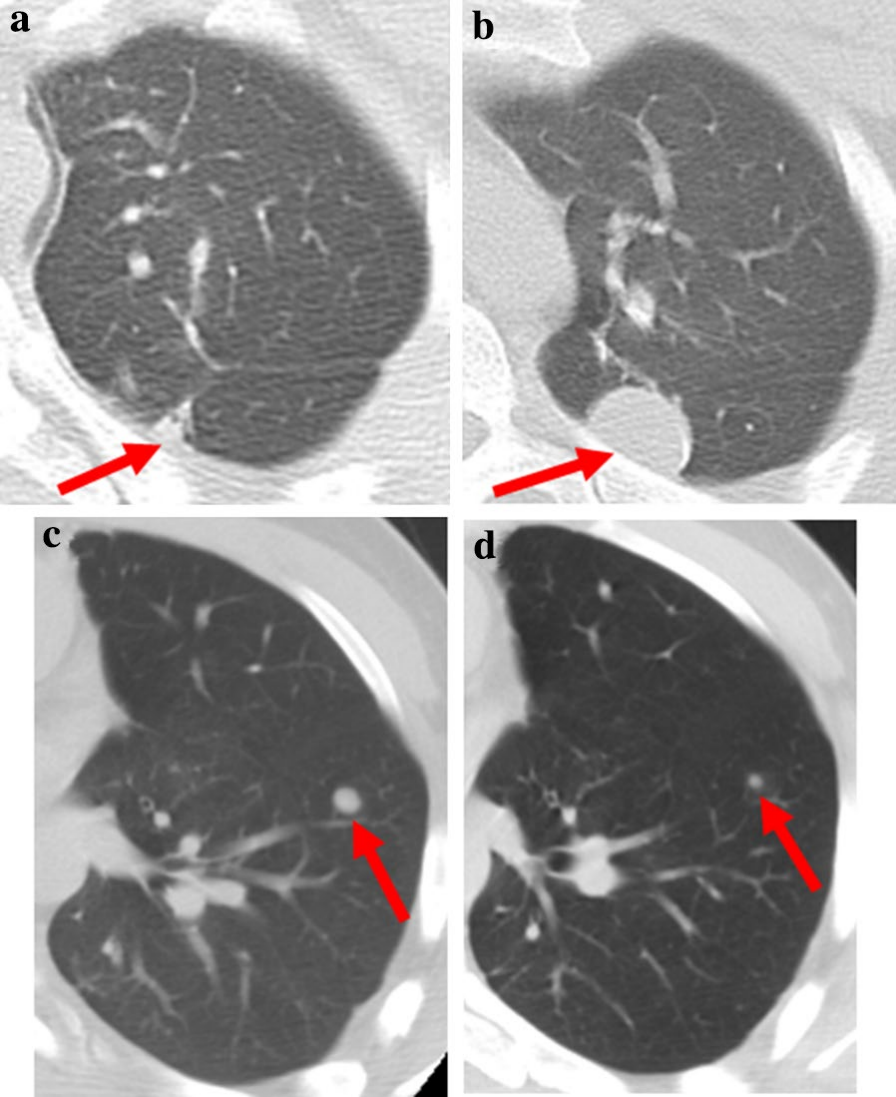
a Axial CT scan performed on 6/10/10 shows a new subpleural 6 mm *left*
